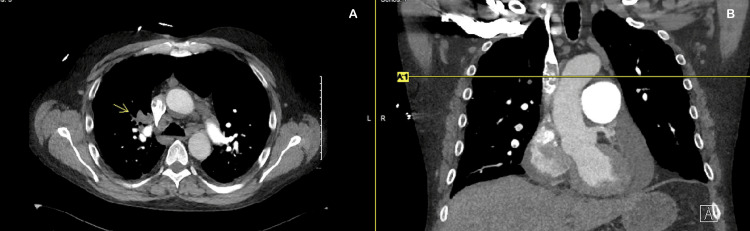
Right upper lobe pulmonary embolism, right pulmonary artery occlusion (yellow arrow) A: CT pulmonary angiogram, bilateral pulmonary emboli; B: venogram, extensive femoropopliteal DVT. DVT: deep vein thrombosis
